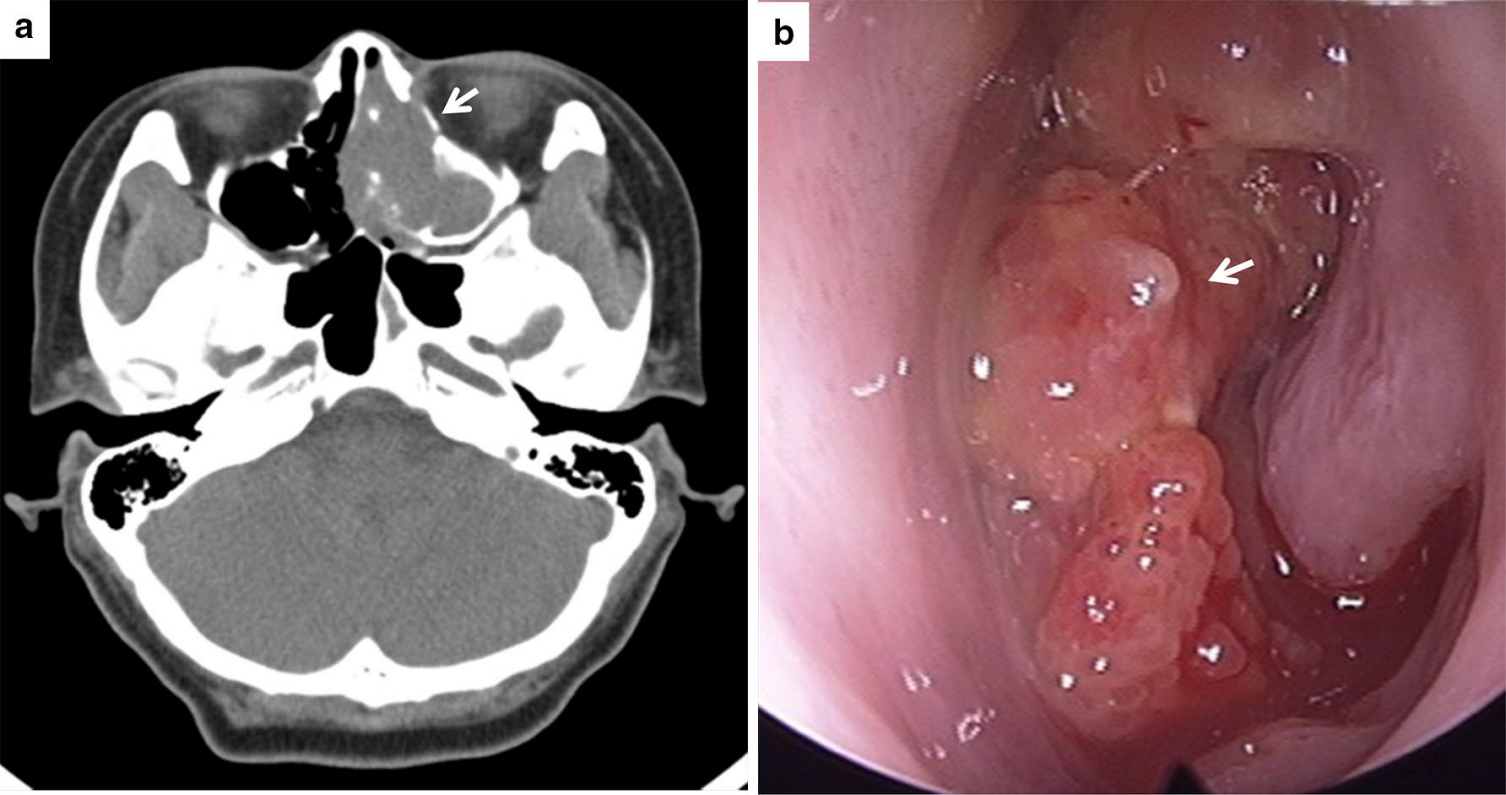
a Computed tomography showing a left nasal mass (*arrow*) involving the middle and inferior turbinate of the left nasal cavity as well as the medial wall of the left maxillary sinus, the lateral wall of the left maxillary sinus, and the left ethmoidal sinus and causing septal deviation. b A bulging mass (*arrow*) on the nasal septum was noted on nasal endoscopy
